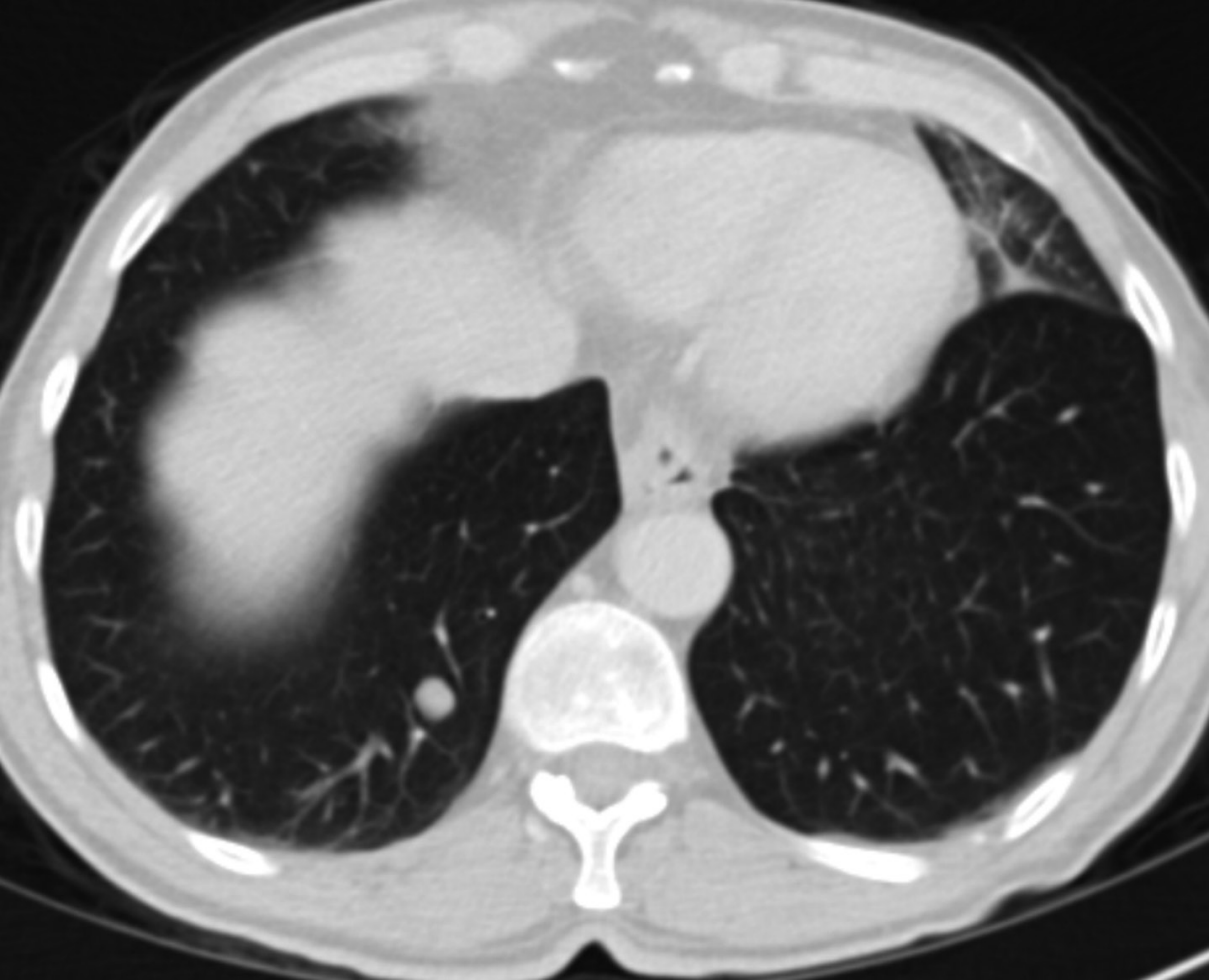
An axial chest CT section of a 57-year-old male patient shows an 11 × 10 mm nodule in the right lower lobe posterobasal segment. CT, computed tomography.
